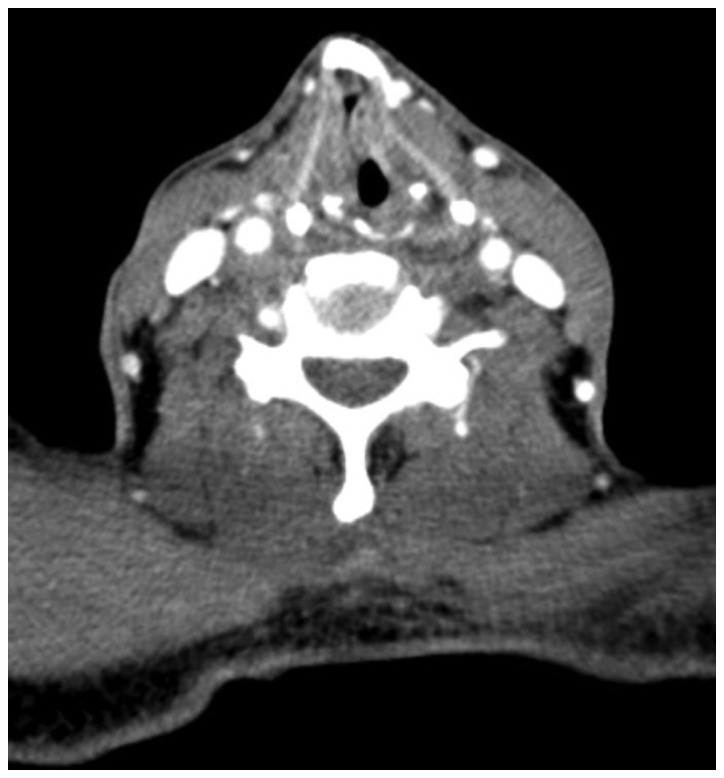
Computed tomography findings of case two showed a 1.5×1.0-cm mass on the left vocal cord.
